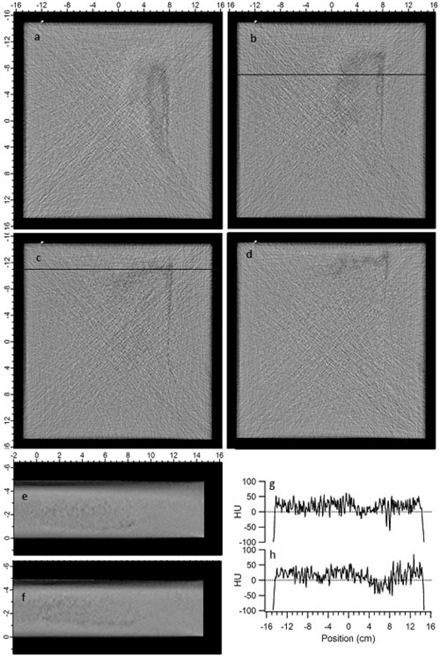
CT images of Sample B showing representative density variations (a)–(d) in the plane of the slab, and in the cross section (e) and (f) at the location indicated by the horizontal lines in (b) and (c), respectively. Line profiles (g) and (h) are shown from (b) and (c), respectively, as indicated by the horizontal lines.
